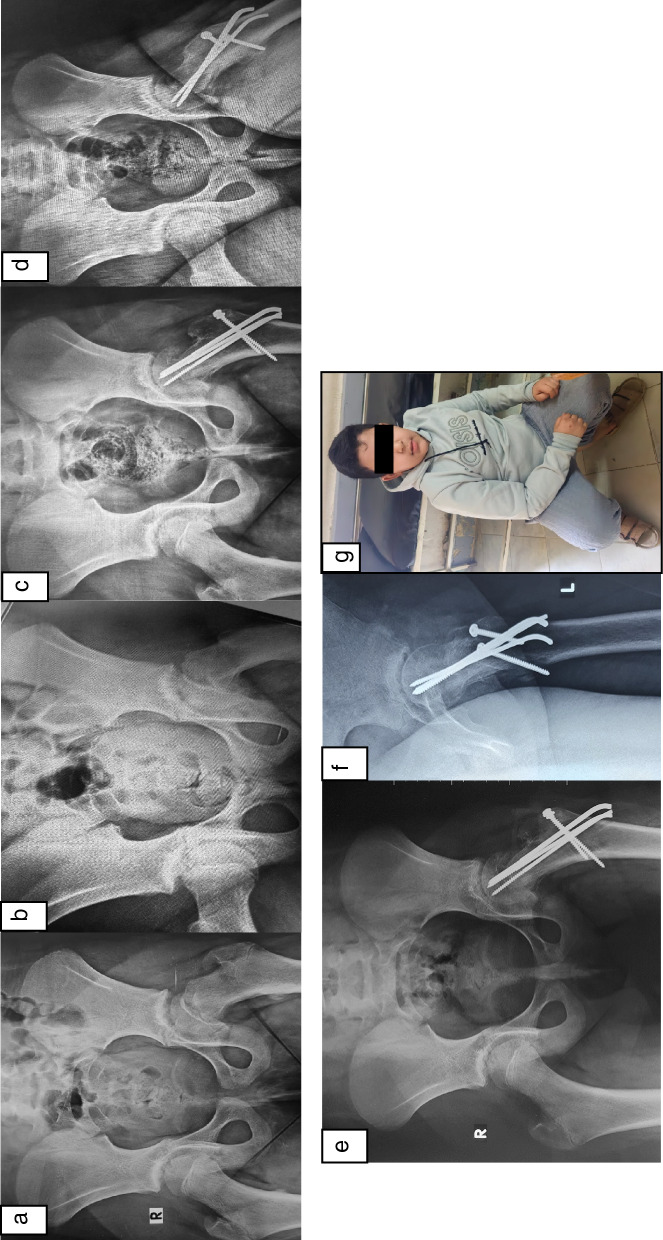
a, b Preoperative radiographs (ap and lateral) showing left SCFE in 10-year-old boy. c, d Postoperative radiographs (ap and lateral) follow-up at 1-year follow-up after MDP and fixation by three Schanz screws (3 mm). e–g Postoperative radiographs (ap and lateral) at the final follow-up visit (7 years) and photo showing excellent normal left hip ROM and function
